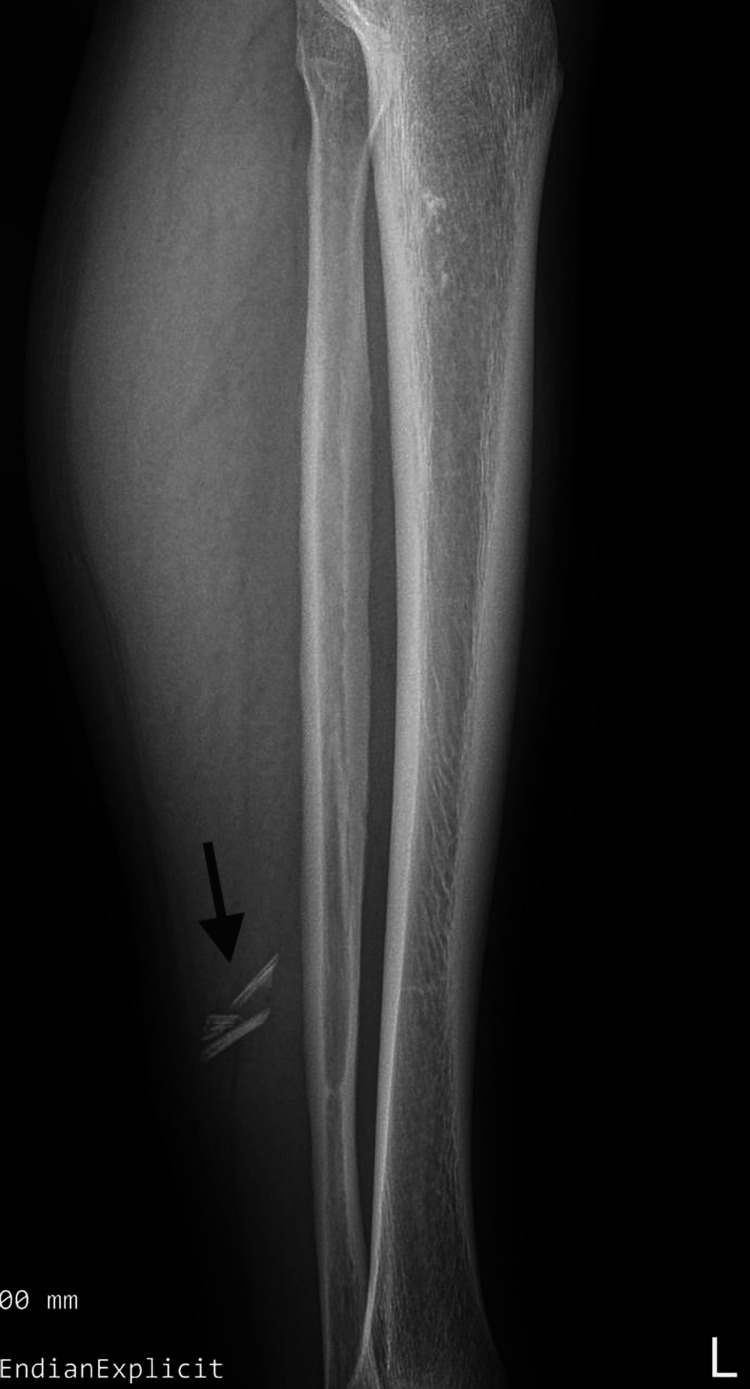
Plain radiograph of the left leg demonstrating multiple linear radiopaque foreign bodies (black arrow) within the soft tissue.
